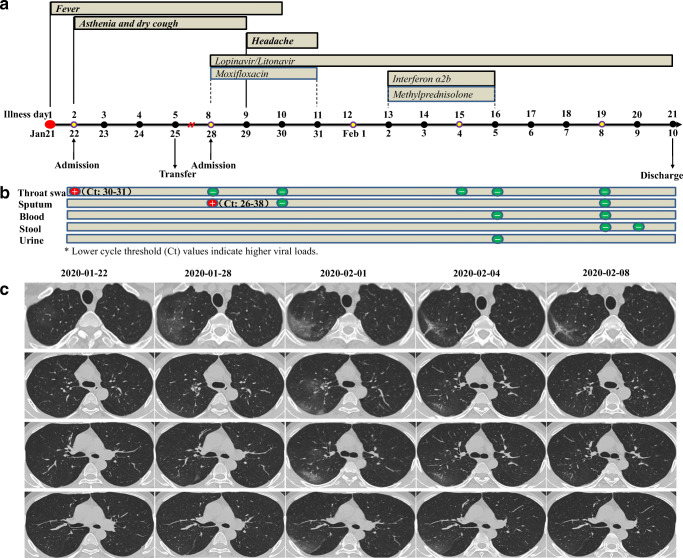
The symptoms (a), 2019-nCoV-RNA detection (b), and dynamic CT scan (c) according to clinical course of disease. The 2019-nCoV-RNA was quantified by real-time RT-PCR. The primers and probe targeting to ORF1ab and N gene were used. Lower cycle threshold (Ct) values indicate higher viral loads, and Ct values more than 40 indicate indeterminate
